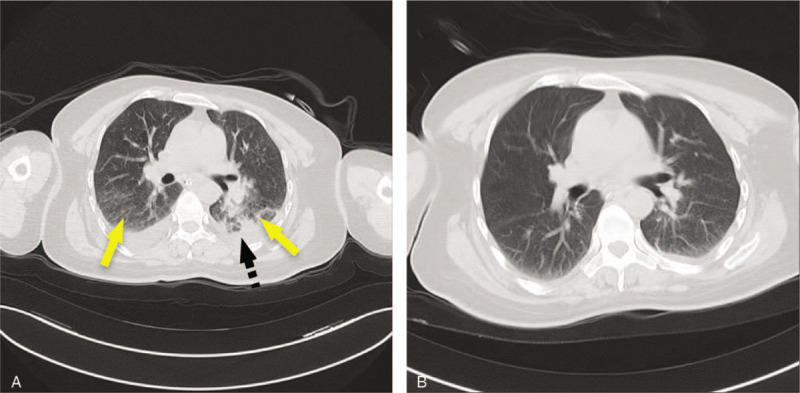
Axial nonenhanced lung CT: (A) showing bilateral involvement with excavation (black arrow) and pleural effusion (dashed arrow) (B) showing improved lesions.
